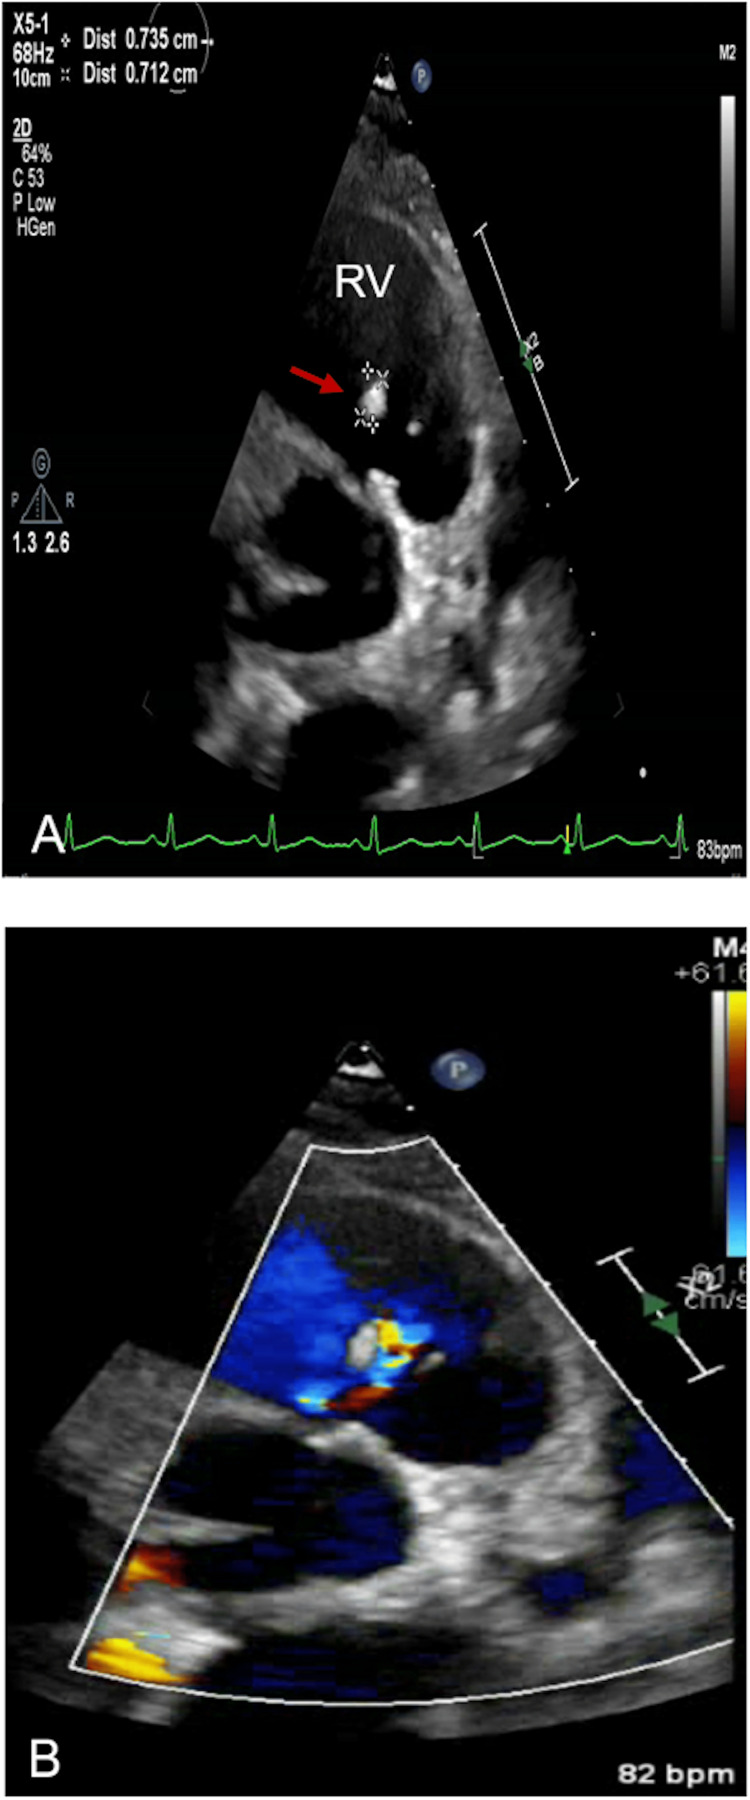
(A) 7 mm × 3 mm vegetation on the pulmonic valve (arrow). (B) Color Doppler of the pulmonic valve. RV: right ventricle.
